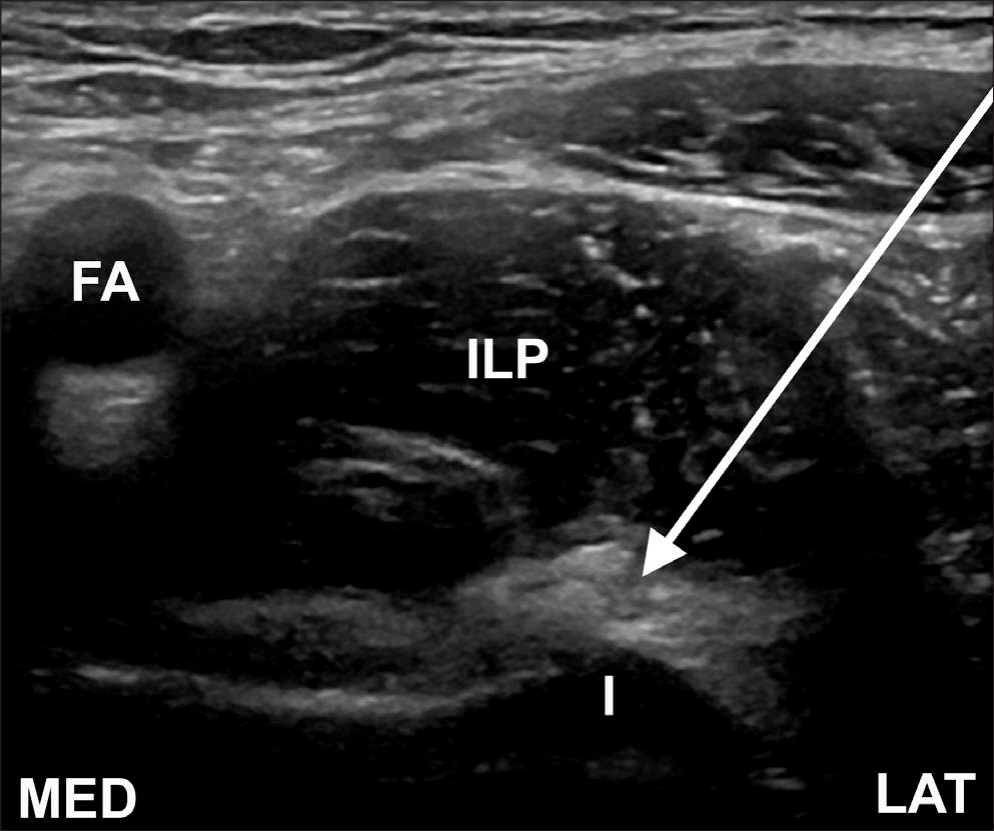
Transverse oblique ultrasound image superior to the femoral head, demonstrating the iliopsoas tendon. The needle is directed between the deep surface of the iliopsoas tendon and the superficial surface of the ilium (I) from a lateral approach (arrow) at the level of iliopectineal eminence. FA, femoral artery; ILP, iliopsoas muscle; LAT, lateral; MED, medial.
